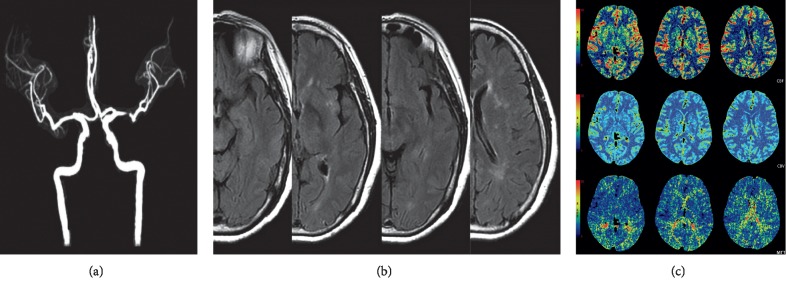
FVH absent in a patient with left middle cerebral artery severe stenosis (M1). (a) MRA showed a severe stenosis of left MCA M1. (b) No hyperintense signal of the middle cerebral artery branch was found in the corresponding fissure or the sulci of the left fronto-parietal lobes. (c) CT perfusion imaging showed hypoperfusion with prolonged MTT in the bilateral anterior and posterior cortical border zones, and no clearly decreased CBF or CBV values were found.
